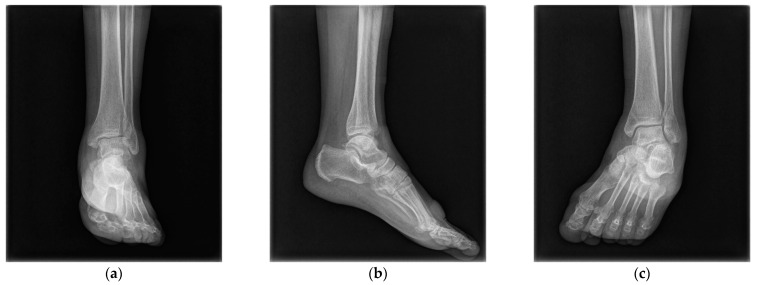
Preoperative anteroposterior (a), lateral (b), and mortise (c) X-ray images of a 47-year-old woman’s left ankle, revealing a short oblique fracture of the lateral malleolus and a Chaput fracture.
